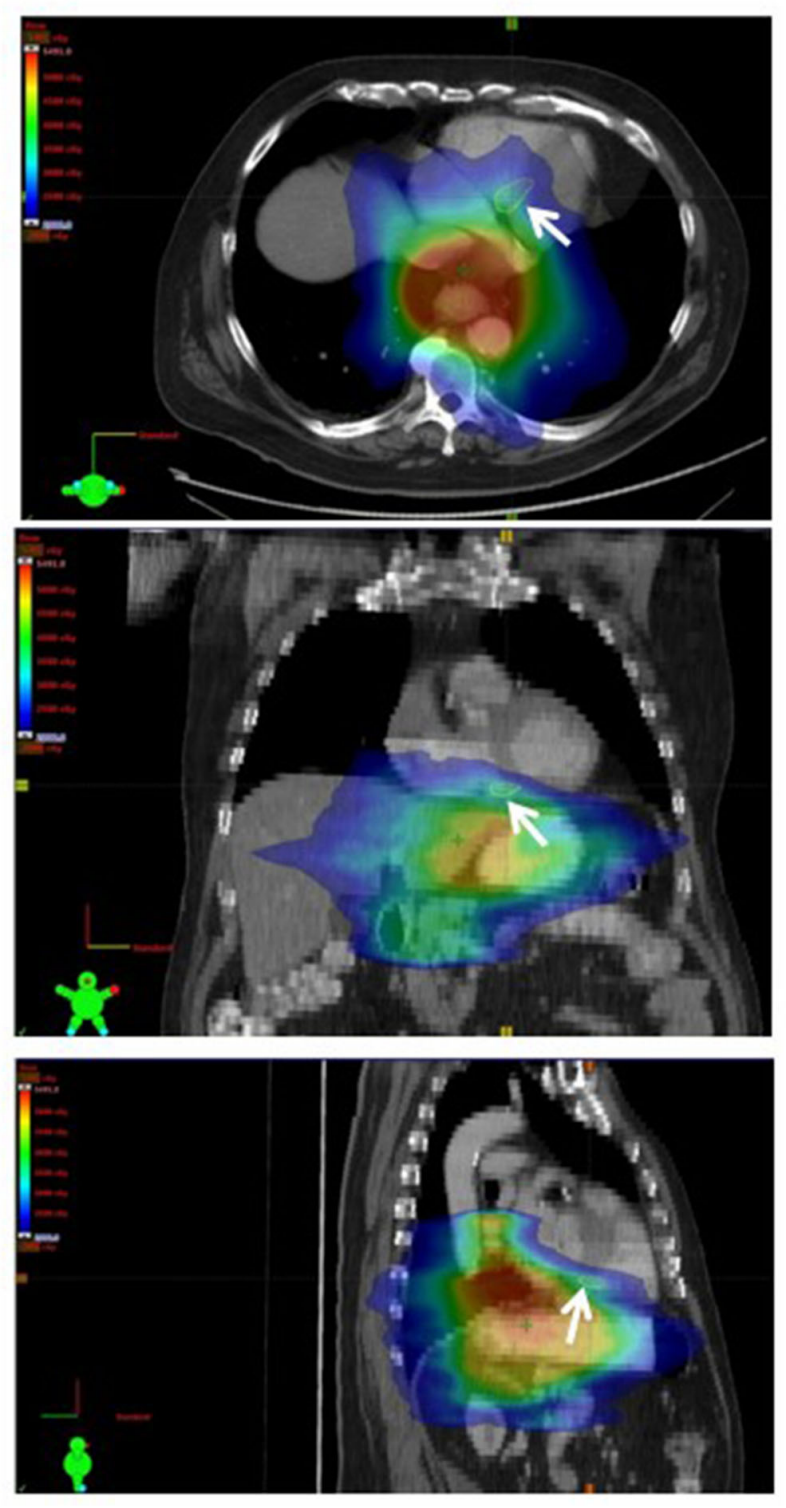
Representative treatment plan with axial (top), coronal (middle), and sagittal (bottom) CT images with color-washed dose distribution. The basal septal region is delineated (white arrow).
